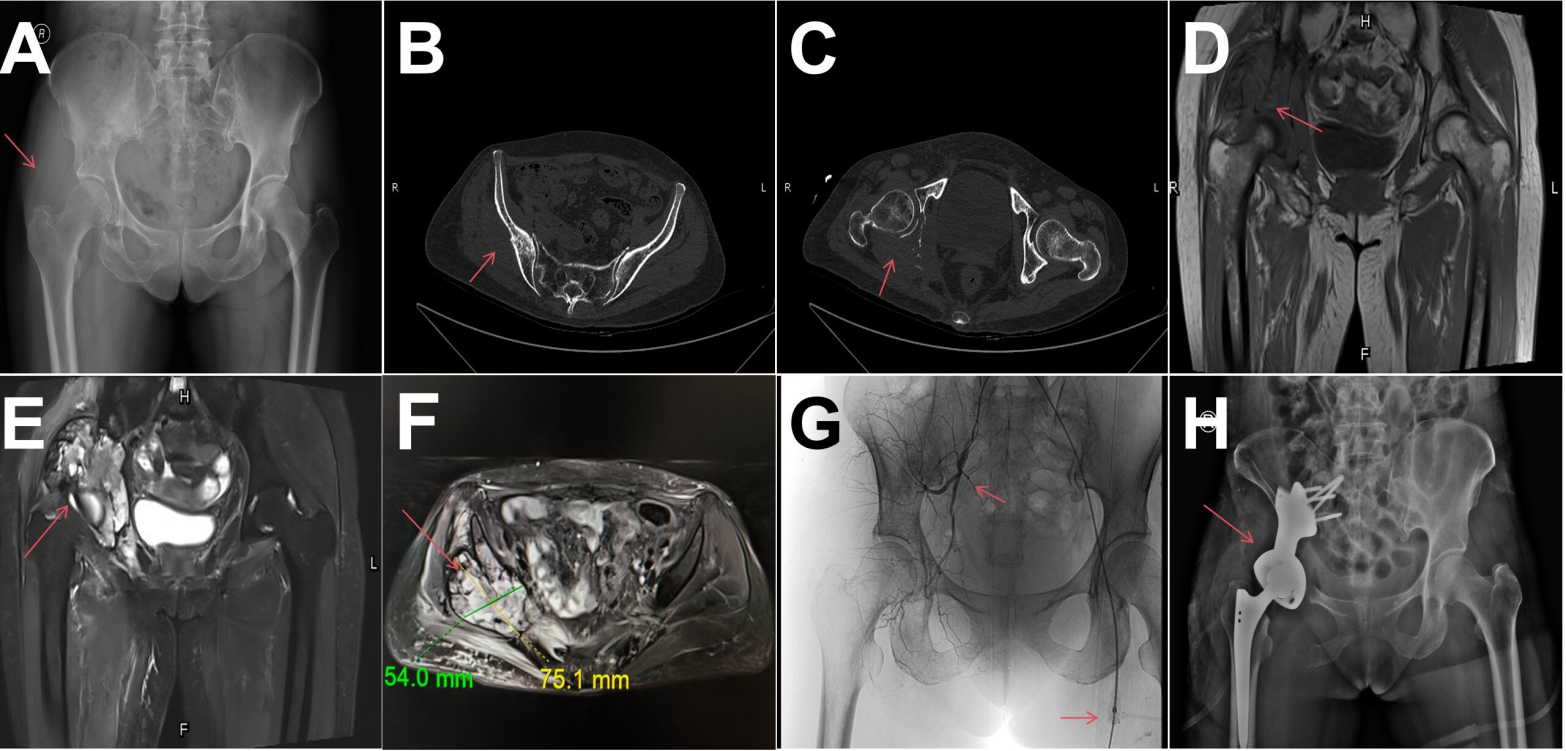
Post-chemotherapy imaging and surgical intervention. (A-F) X-ray, CT and MRI after chemotherapy: showed a significant reduction in tumor volume compared to preoperative images. (G) The vascular supply to the tumor was revealed by DEB-TACE. (H) X-ray after operation: the fixation of the 3D-printed prosthesis is satisfactory, showing no signs of loosening.
